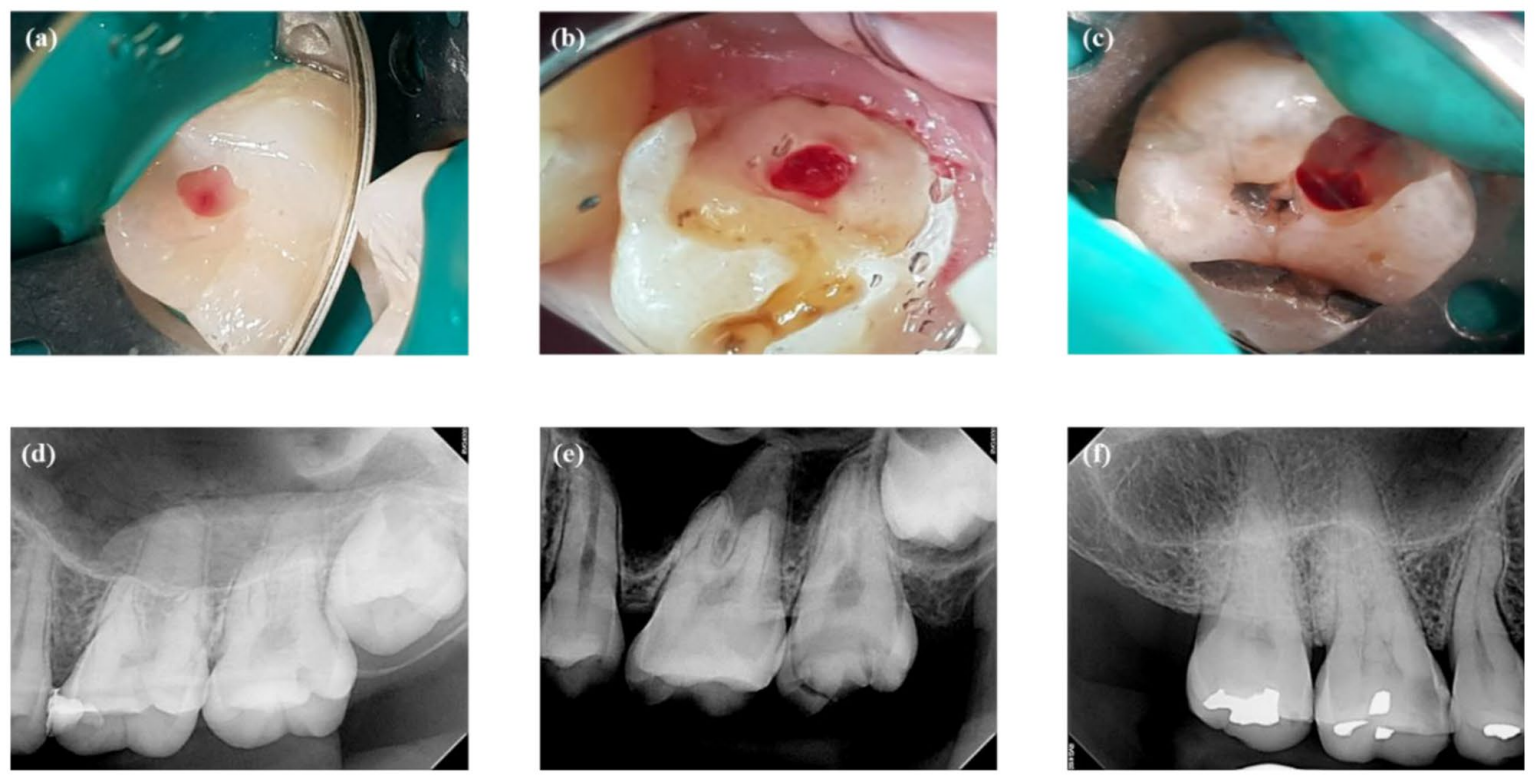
Clinical photos of pulp exposure sites and periapical radiographs. (a) Pulp exposure after removal of the secondary caries beneath the old resin restoration in case (1) (b) Pulp exposure after caries removal in case (2) The loss of tooth material is severe, and crown restoration is required. (c) Pulp exposure after caries removal in case (3) The size of the pulp exposure site and extent of tooth decay are larger than those in case 1. (d, e, f) No radiographic changes can be observed in the periapical regions
